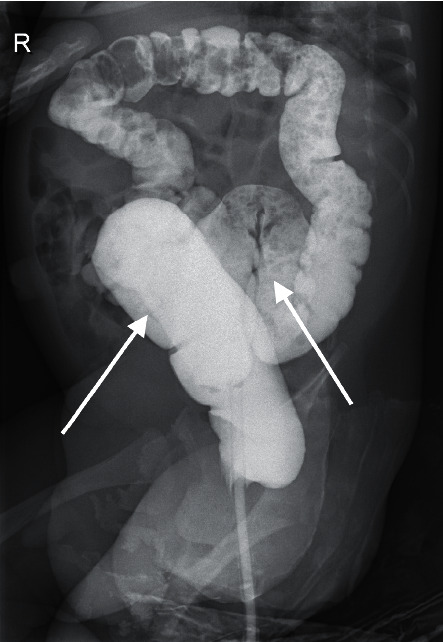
Barium enema X-ray. Dolichocolon (additional curves at sigmoid and transverse colon, arrows), no strictures, no pathological bowel dilatations, and no signs of Hirschsprung's disease.
